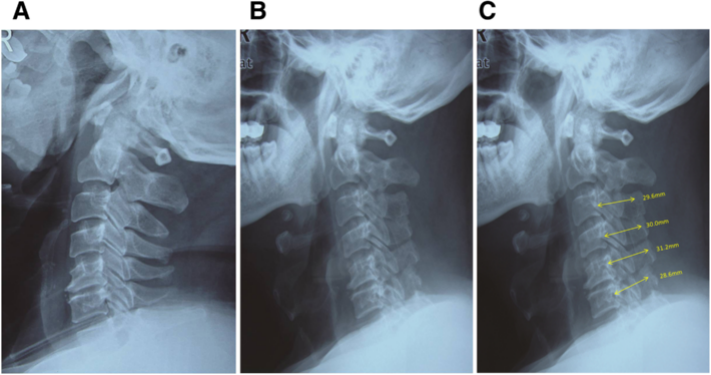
Lateral radiographs showing expanded canal. A Preoperative radiograph. B Immediate postoperative radiograph demonstrating elevated laminas. C Postoperative radiograph demonstrating the measurements of anteroposterior diameters of elevated laminas.
